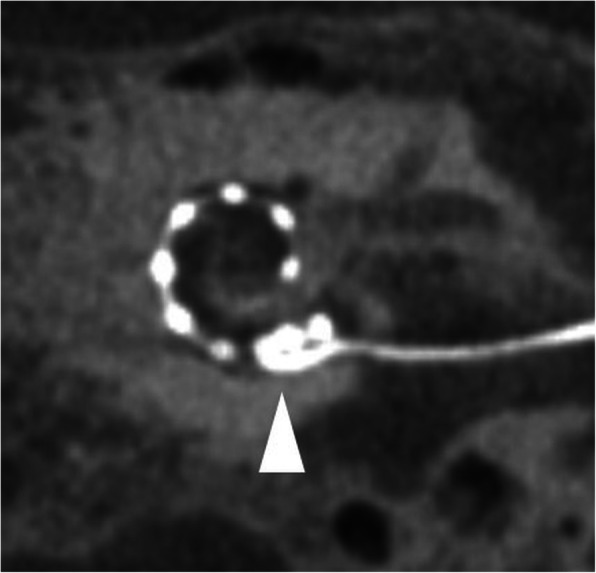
A 55-year-old female patient, with basal fold-over. CBCT paraaxial maximum intensity projection image shows fold-over of the basal part of the electrode within the cochlea (arrowhead)
